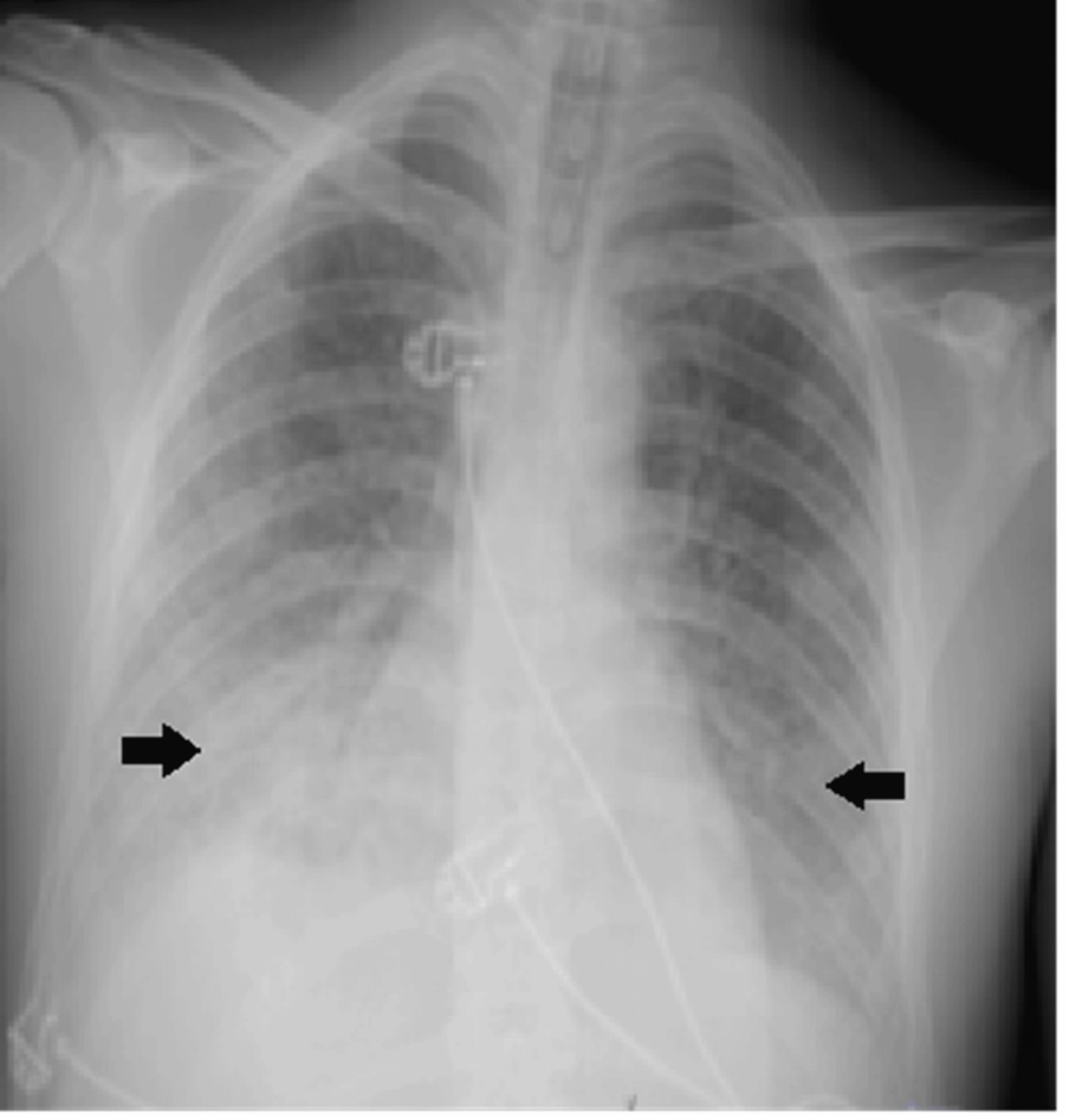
Control chest radiograph showing improvement in lung infiltrates
